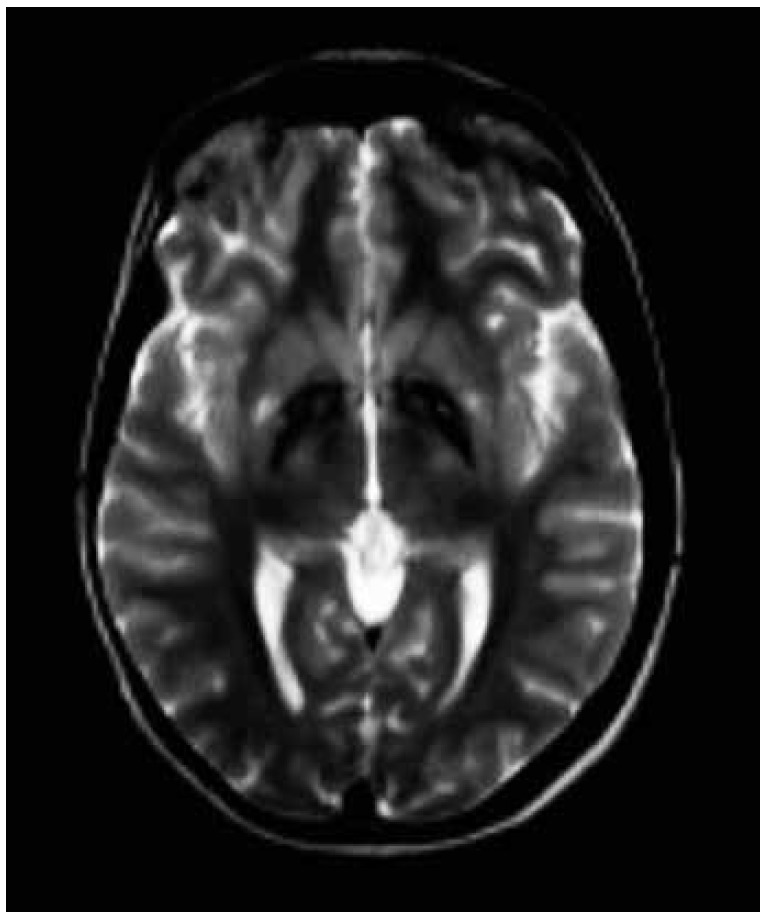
T2-weighted magnetic resonance imaging showing symmetrical central hyperintensity surrounded by hypointense signal in globus pallidus, giving "eye of tiger" appearance.
